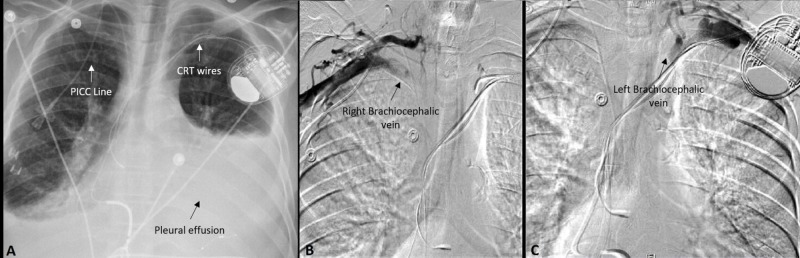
Imaging prior to intervention Large left-sided pleural effusion seen on chest x-ray (A) prior to angioplasty. Right-sided PICC (peripherally inserted central venous line) and left-sided CRT (Cardiac resynchronization therapy) wires can be appreciated as well. Occlusion of the right (B) and left (C) brachiocephalic veins can be seen on the venogram.
